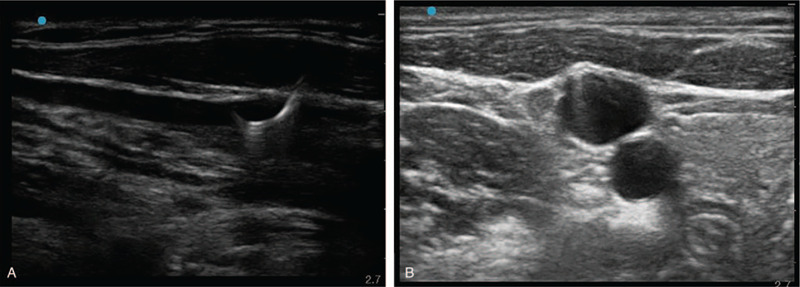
Broken J tip of the guidewire was withdrawn using real-time ultrasound guidance. Coil filament outside of the core wire was still connected.
